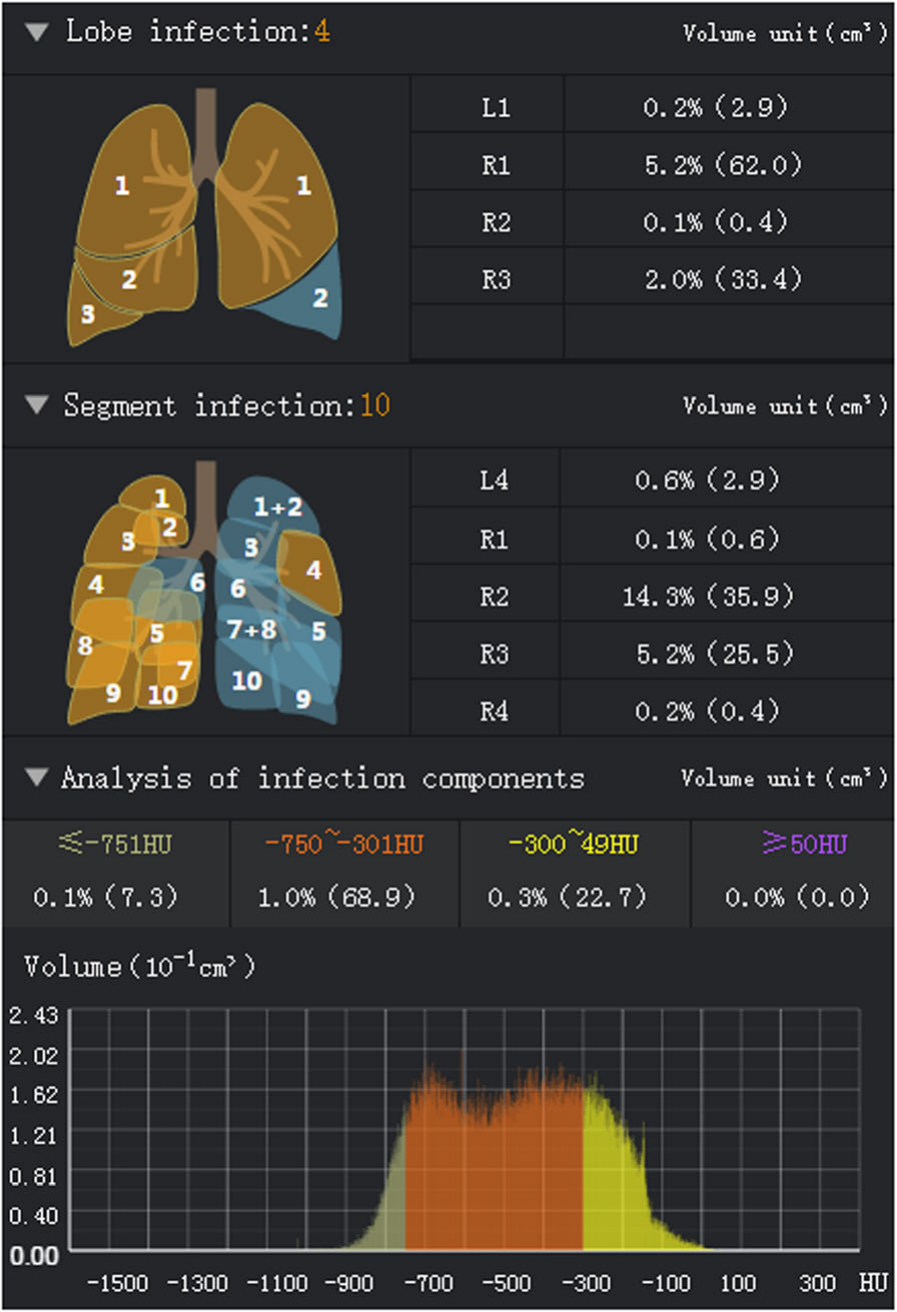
Software interface obtained by inputting original HRCT images of one patient into the DL system. The VOIs and POIs in the lung lobes and bronchopulmonary segments are presented; the HU histogram within the infection regions can be visualized
